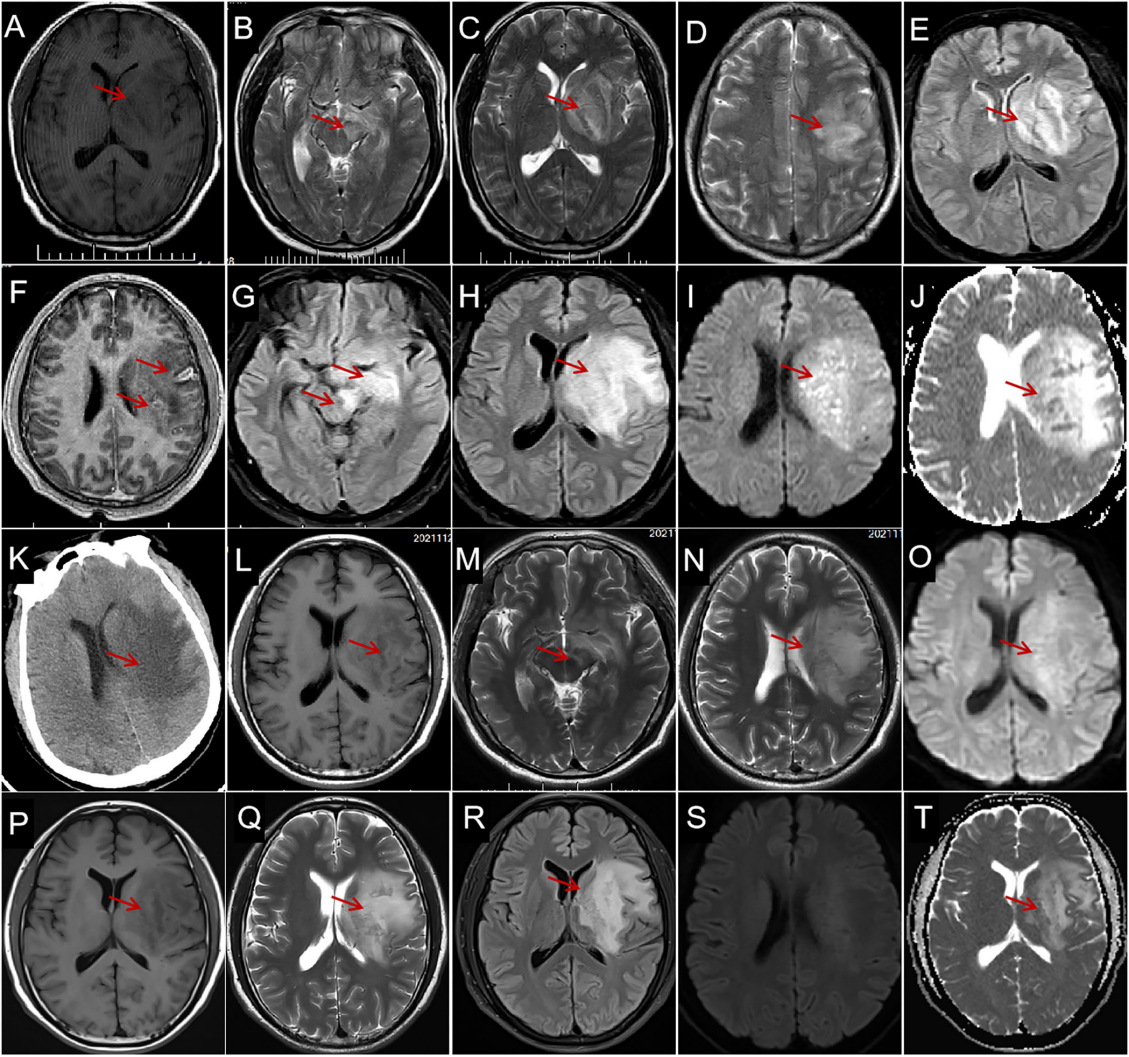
Characteristics and evolution of lesions on brain magnetic resonance imaging (MRI) and computed tomography (CT). (A) Brain MRI on day 1 shows a large hypointensity (arrows) in the left basal ganglia and the temporal lobe on axial T1-weighted imaging (WI); a hyperintense region (arrows) in the left midbrain (B), basal ganglia (C), and temporal-parietal lobe (D) on T2-WI and fluid-attenuated inversion recovery [FLAIR (E)] with space-occupying effect; and individual, small, arc-shaped, ring-shaped lesions are enhanced [(F), arrows]. The MRI on day 5 shows extensive hyperintense lesions enlarged (arrows) in the left midbrain, hippocampus [(G), arrows], basal ganglia, and temporal lobe [(H), arrows] on FLAIR, diffusion-weighted imaging [DWI (I), arrow], and apparent diffusion coefficient [ADC (J), arrow]. CT on day 6 reveals extensive, finger-shaped, low-density areas with space-occupying effects in the left basal ganglia and semioval center [(K), arrow]. MRI on day 34 shows slight hypointensity on T1-WI [(L), arrow], slight hyperintensity on T2-WI [(M,N), arrows], and DWI [(O), arrow], and the space-occupying effect obviously improved. Magnetic resonance imaging on the 25th day after discharge shows hypointensity on T1-WI [(P), arrow], hyperintensity on T2-WI [(Q), arrow], FLAIR [(R), arrow] ADC [(S), arrow], and without diffusion restriction on DWI (T).
